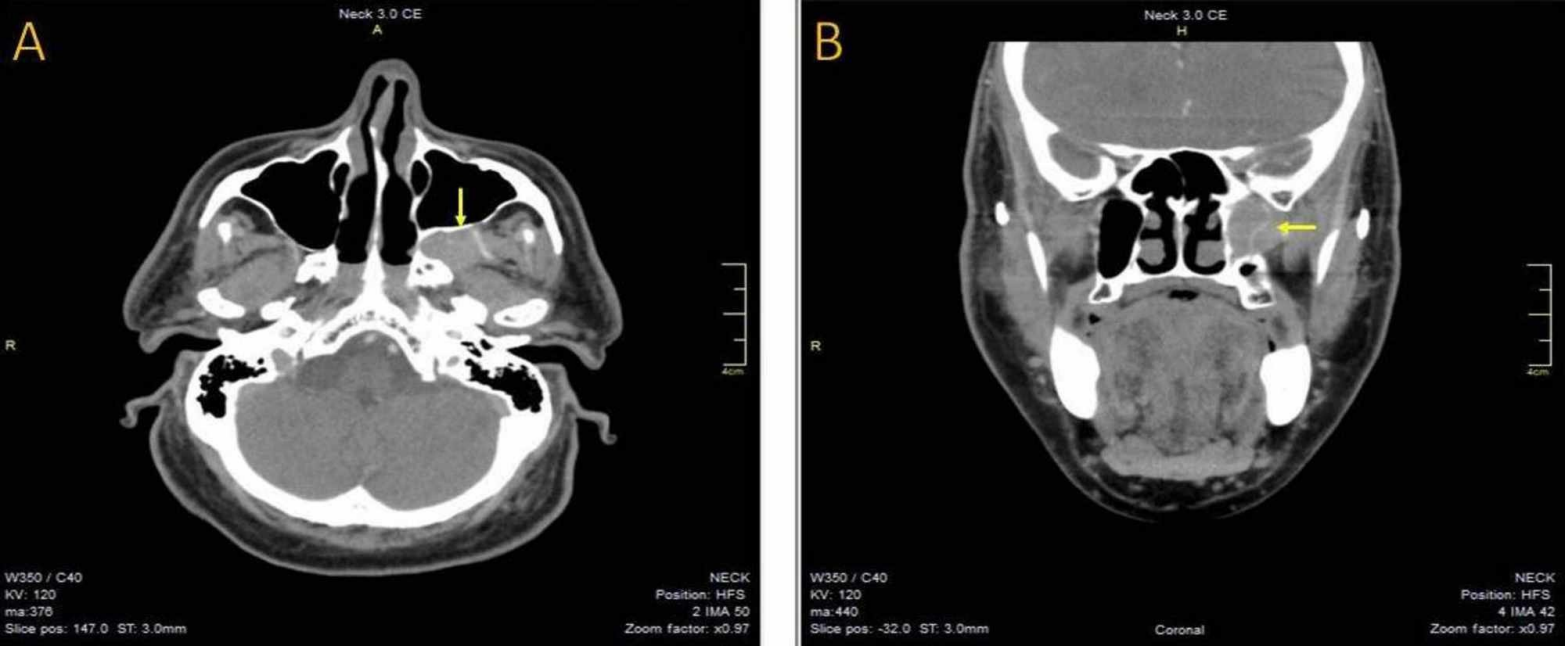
(A) and (B) The axial and coronal views of a CT scan of the sinuses demonstrating an imprint on the posterior wall of the maxillary sinus due to compression.
